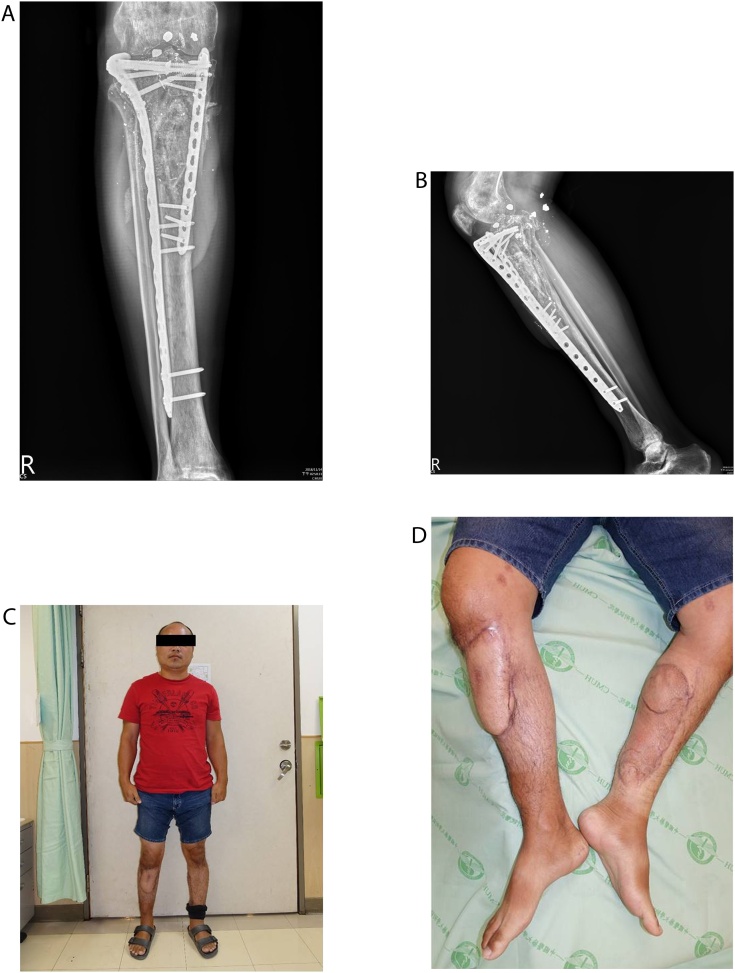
(A) Radiograph at 12-months-follow-up, AP view and (B) lateral view (C) Patient is able to stand without leg length discrepancy (D) Well-healed skin graft and flap on both lower extremities.
